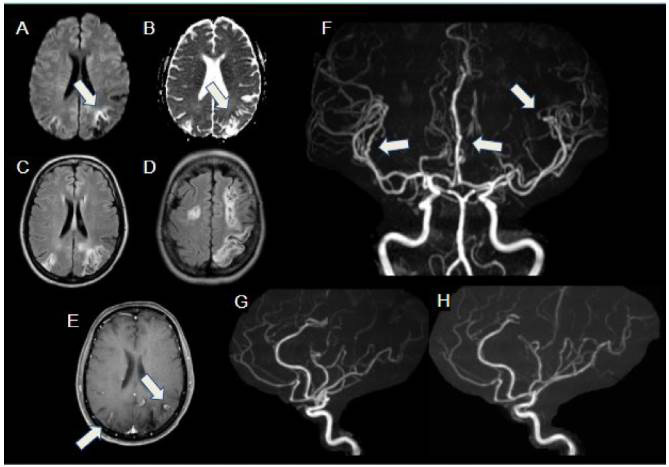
Systemic lupus erythematosus vasculitis. Multiple infarctions affecting different vascular regions, in various stages of healing in a 14-year-old female patient presenting with right lower limb weakness. Hyperintensity areas on DWI (A) with corresponding low-signal ADC map (B) show restricted diffusion in the left parietal region, in the anterior border of a previous infarct, compatible with acute stroke (arrows). Axial FLAIR images (C and D) show multiple cortical-subcortical areas of infarction in different vascular regions of both cerebral hemispheres, predominantly on the left. Axial T1 C+ FS (E) shows cortical areas of contrast enhancement in parietal regions, compatible with subacute / chronic infarctions (arrows). MRA images (F, G and H) depict multiple irregularities and stenosis of multiple distal artery branches (arrows), compatible with vasculitis.
